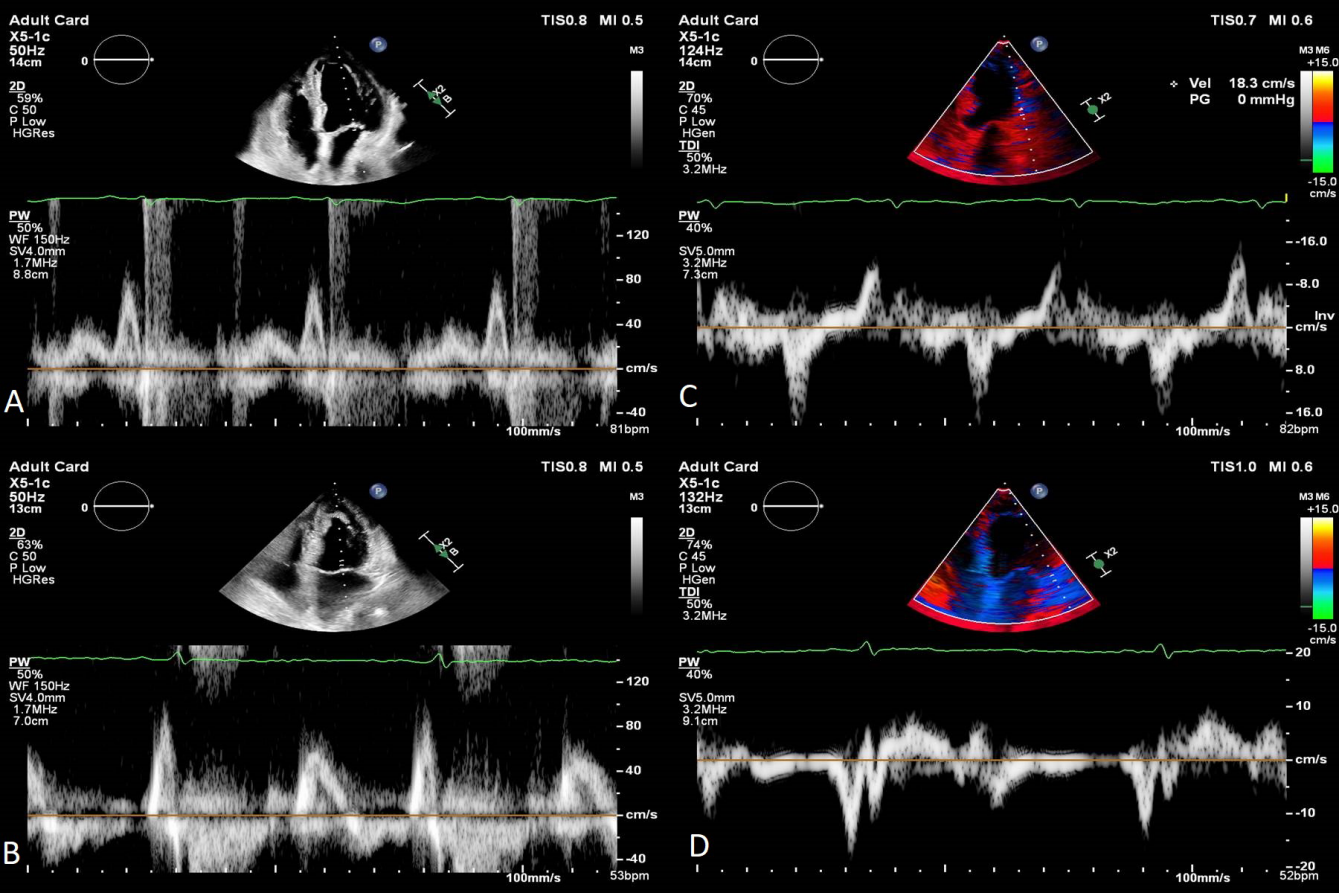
Transthoracic echocardiography images apical four-chambers views
